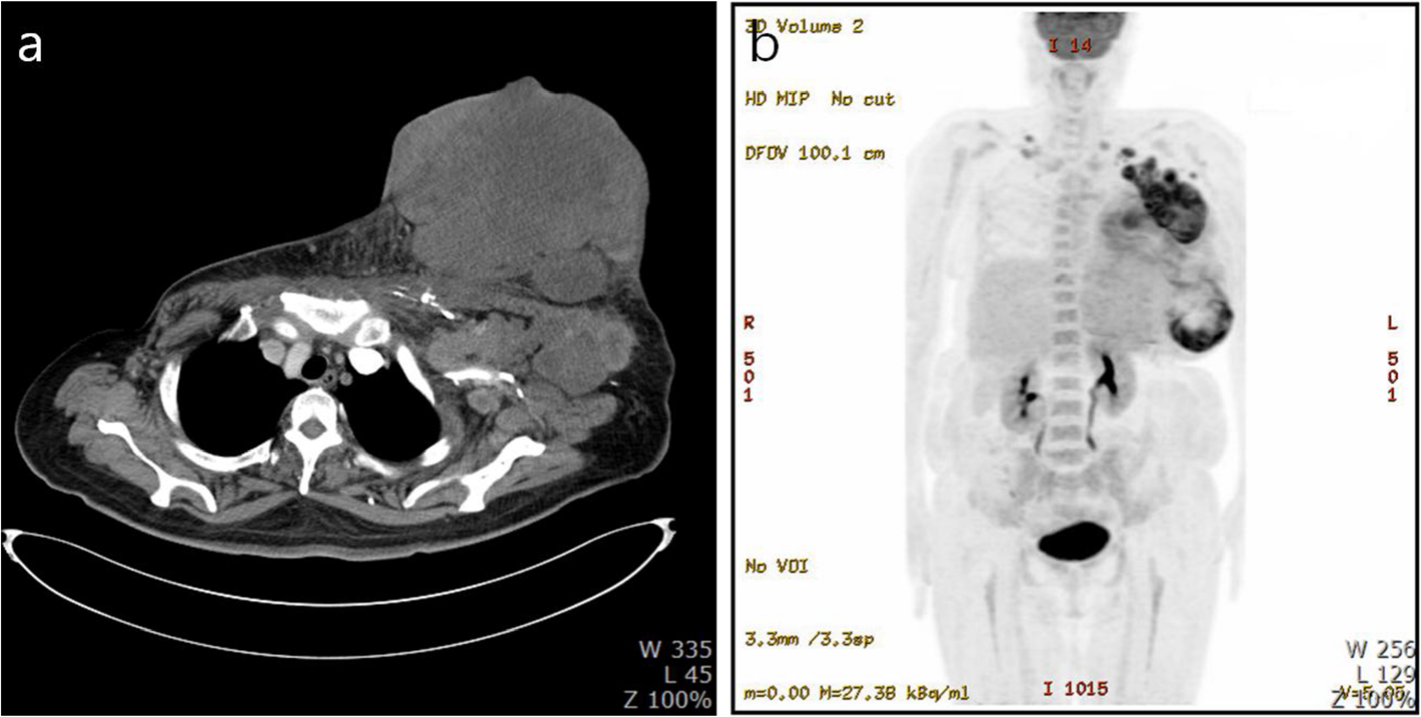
Image findings. (a) Enhanced chest computed tomography (CT) showing a huge breast mass with necrosis and multiple lymph node (LN) enlargements in the left axilla, both supraclavicular area. (b) 18 F-FDG positron emission tomography (PET)/CT revealed a huge uneven hypermetabolic mass and massive hypermetabolic LN metastases without the evidence of distant organ metastasis.
